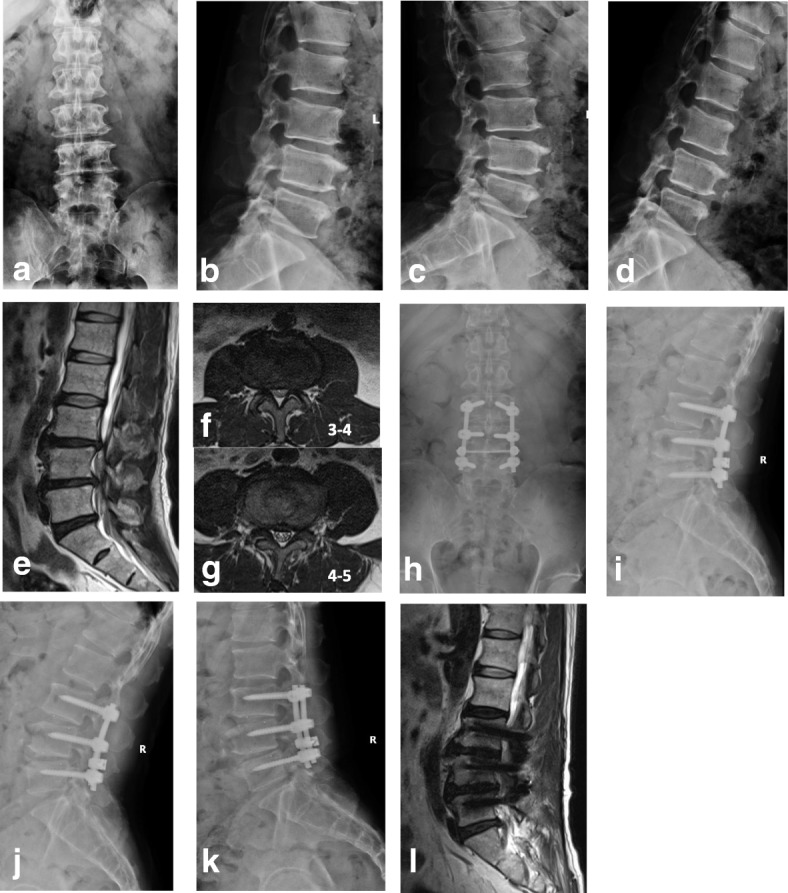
A 66-year-old, male, patient complained of lower back pain and pain in bilateral lower limbs for 2 years and aggravation for 7 months. There was spinal canal stenosis at L3–L5, and the patient received PLIF at L3–L5. a–d X-ray for the lumbar spine in the anteroposterior/lateral and flexion/extension views before surgery; intervertebral mobility 4.3° at L2-L3. e–g Lumbar MRI scan before surgery indicated spinal canal stenosis at L3–L4 and L4–L5, with modified Pfirrmann grade of disc 3 at L2–L3; h–k X-ray for the lumbar spine in the anteroposterior/lateral and flexion/extension views at 36 months after surgery. The intervertebral mobility at L2–L3 was increased significantly to 7.3° after surgery. l MRI scan at 36 months after surgery indicated that the modified Pfirrmann grade of disc was increased to grade 4 at L2–L3
